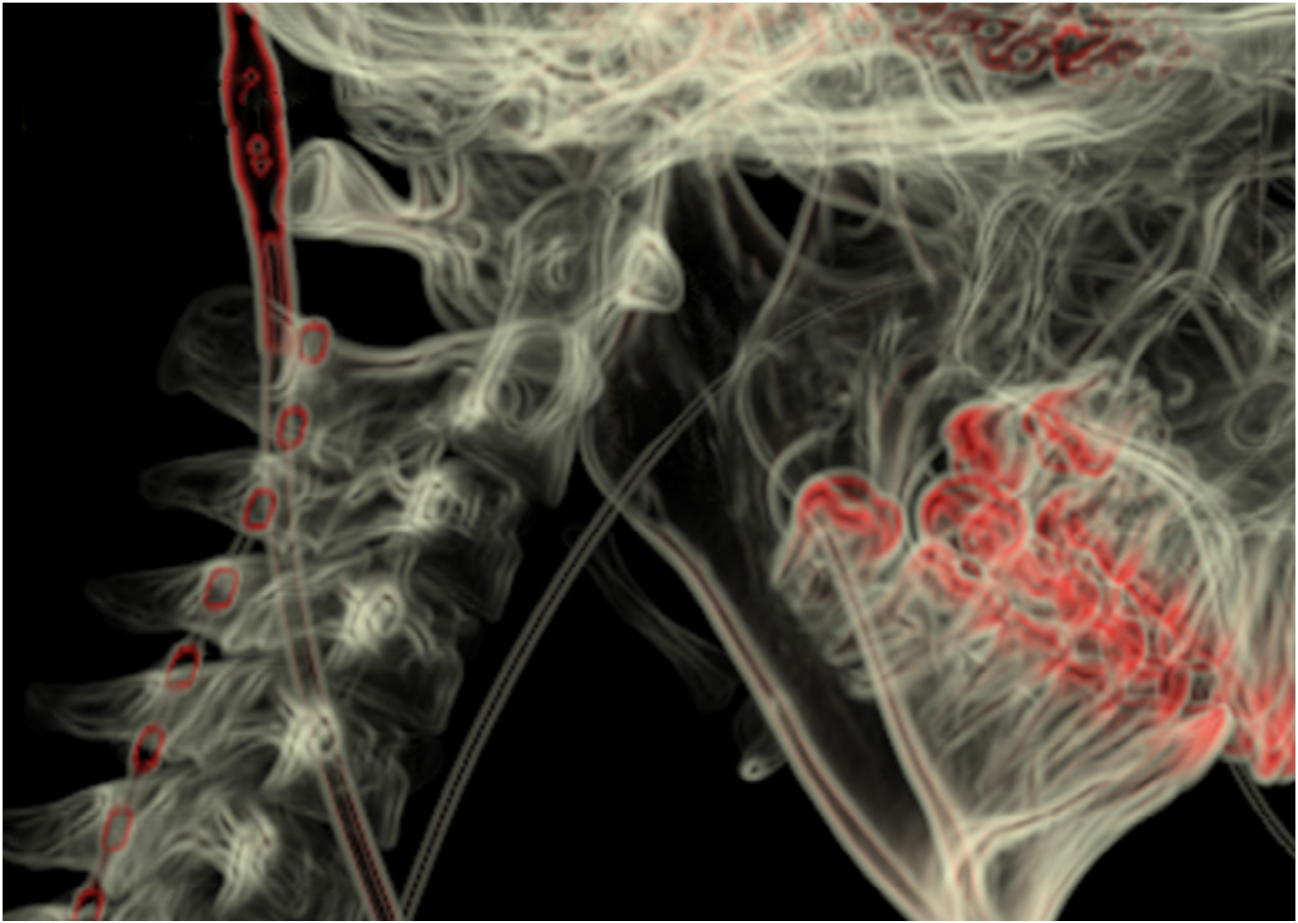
3D reconstruction of cervical CT shows electrode position at epidural space of the C2 vertebrae after short‐term single spinal cord stimulation implantation.
